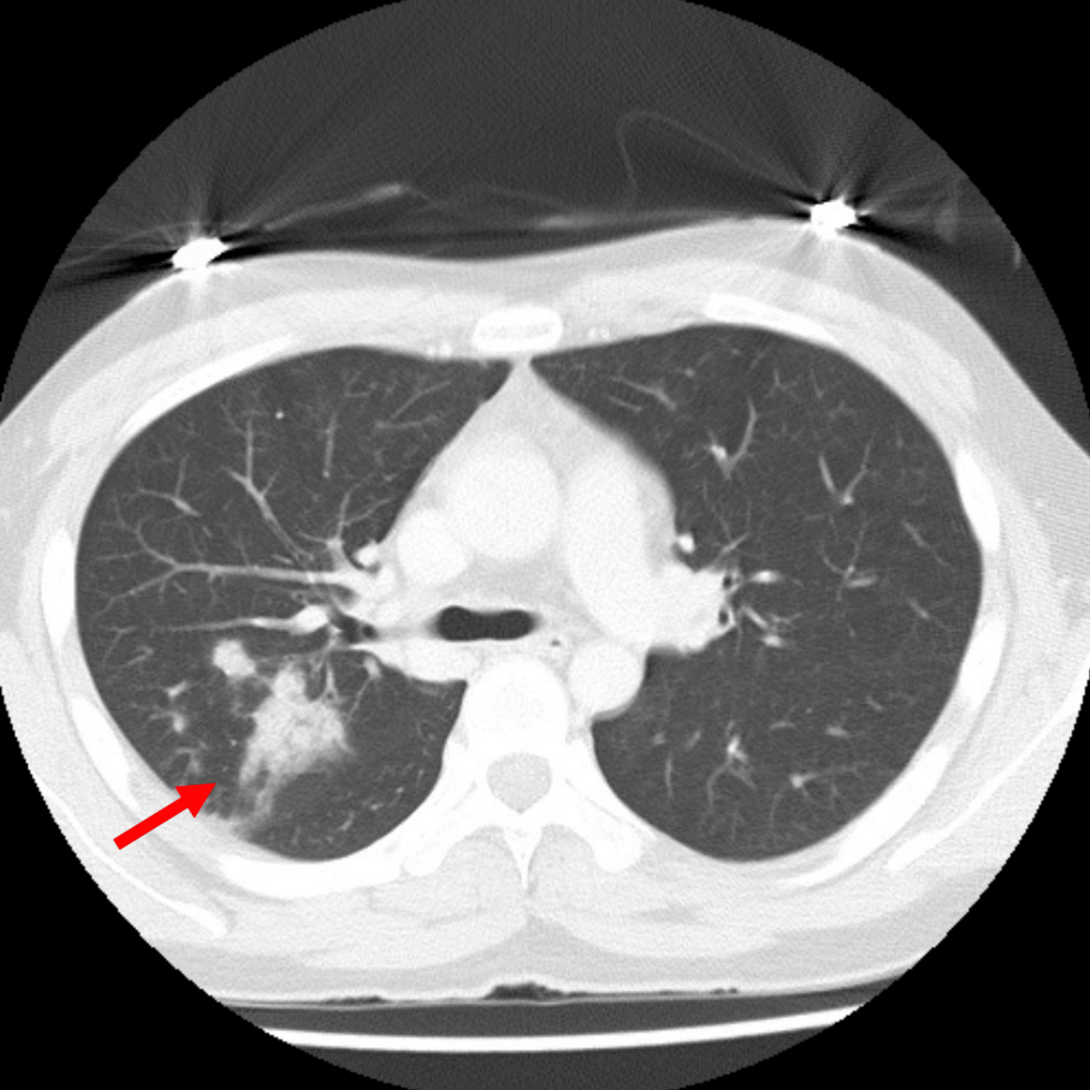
Chest CT on admission showing infiltrates in the right lung (red arrow)
